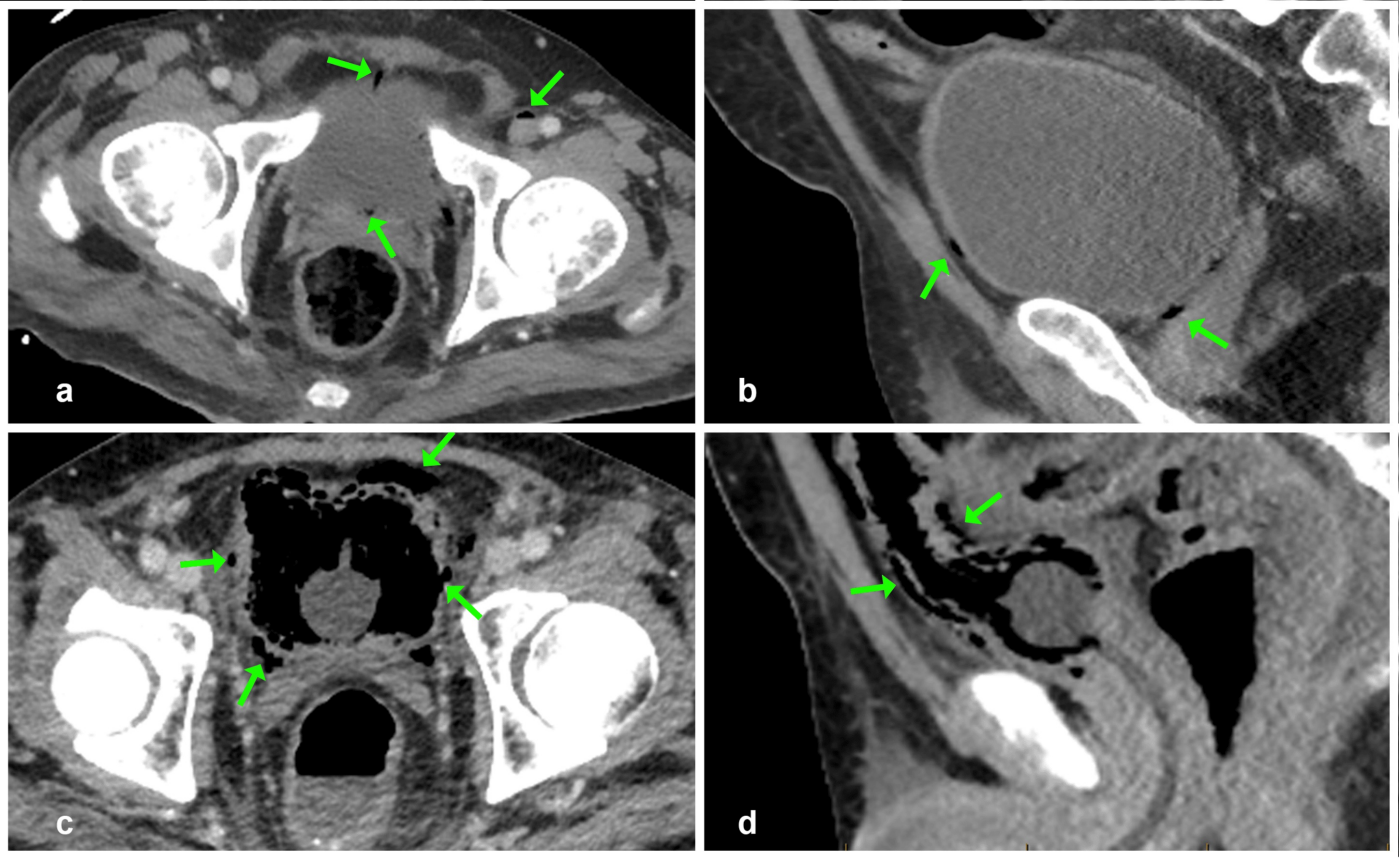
Emphysematous cystitis on CT Initial portal venous phase CT abdomen/pelvis: (a) axial and (b) sagittal views showing linear gas shadow anterior and posterior to the bladder, indicating gas within the pelvic veins. Also, note the presence of gas within the left common femoral vein (green arrows). There is no history of catheterization or attempted line insertion. Figures 1c (axial) and 1d (sagittal) show gas within the bladder lumen and wall, indicating emphysematous cystitis (green arrows).
